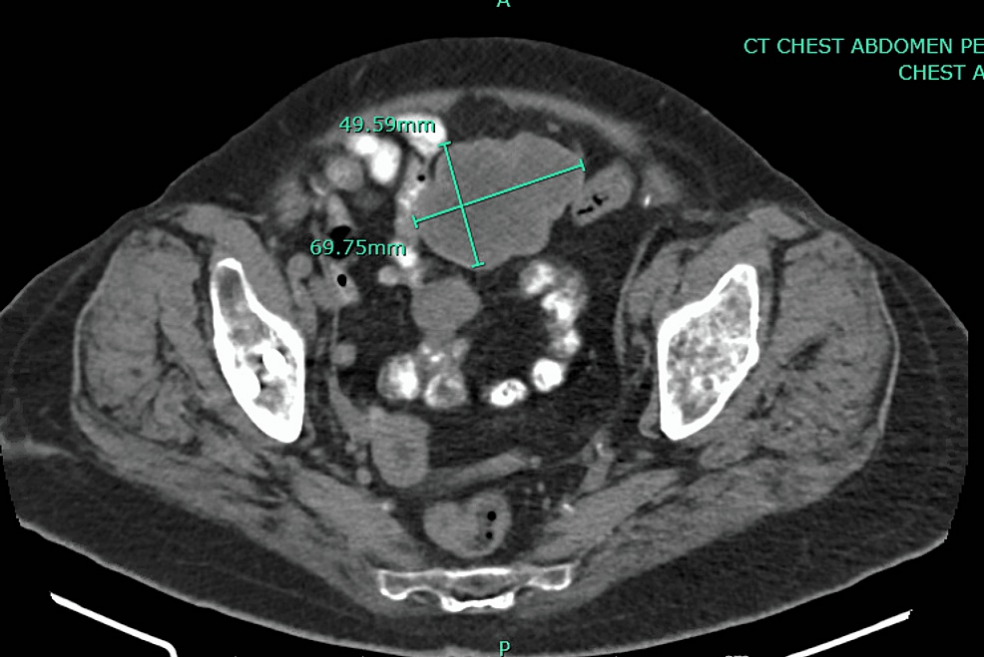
Computed tomography without IV contrast showing regression of primary mass measuring 4.9 cm × 6.9 cm
